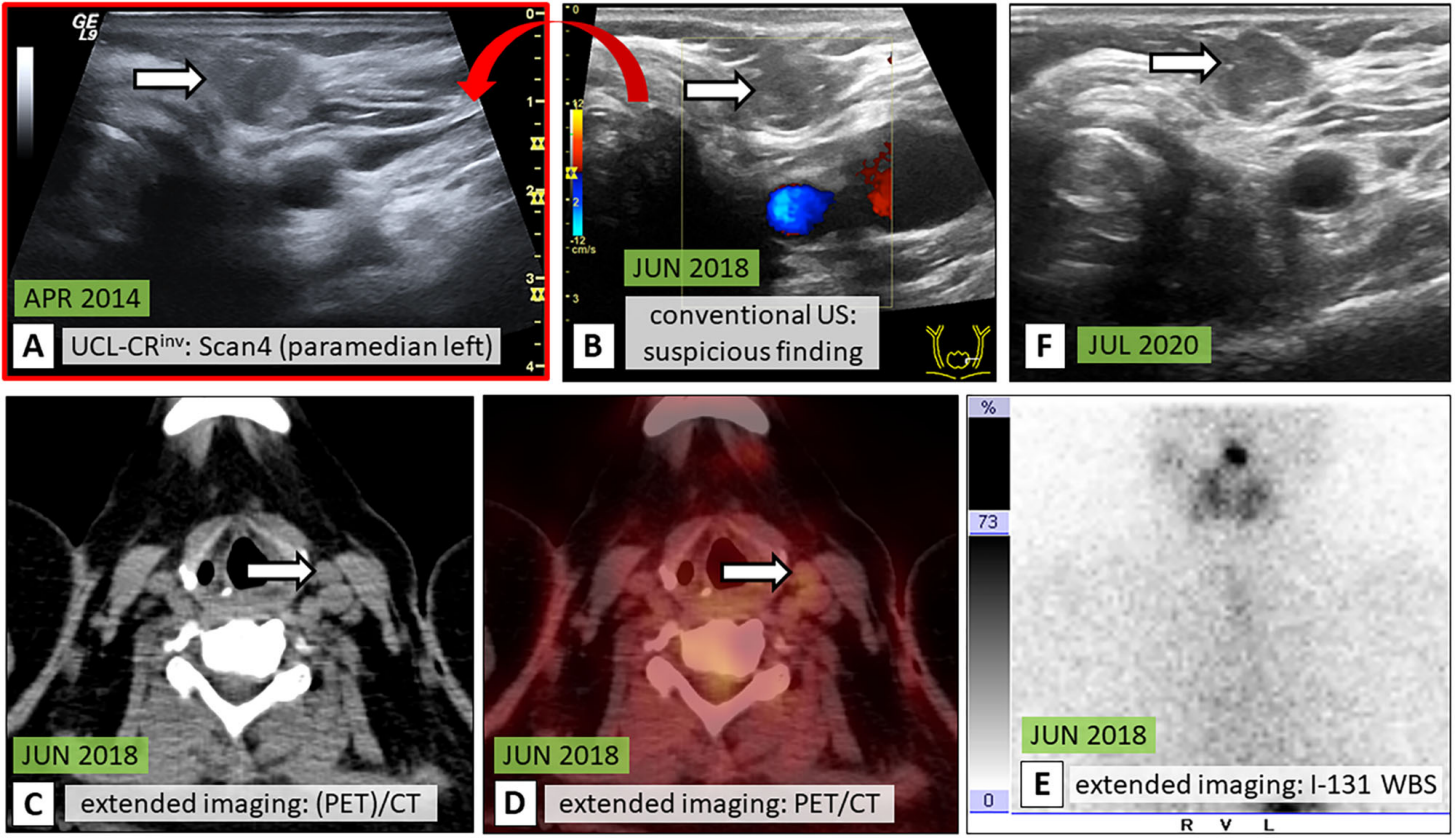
Patient case III: UCL-CR^inv^ with confirmed diagnosis. 49-year-old female at initial diagnosis 2010 (PTC pT2m pN0 cM0). Conventional US revealed a suspicious nodule in the former left-sided thyroid lodge, measuring 9 mm [B, white arrow] after 8 years of FU (JUN 2018). The retrospective PACS review of the UCL from APR 2014 confirmed that the identified lesion is constant over more than 4 years [A, white arrow]. Nevertheless, additional extended imaging was conducted. The lesion was visible on CT [C, white arrow] and did neither show pathological FDG uptake on F-18-FDG PET/CT [D, white arrow] nor pathological I-131 uptake on WBS [E]. The nodule was constant on long-term US FU [F, white arrow]. TG levels were negative throughout the entire time period. UCL-CR^inv^ standardized cervical ultrasound cine loops with clinical relevance—invalidation of a suspicious finding identified on conventional live US, PTC papillary thyroid carcinoma, US ultrasound, FU follow-up, PACS Picture Archiving and Communication System, I-131 WBS iodine-131 whole body scintigraphy, F-18-FDG PET/CT fluorine-18-fluorodeoxyglucose positron emission tomography computed tomography, TG thyroglobulin
